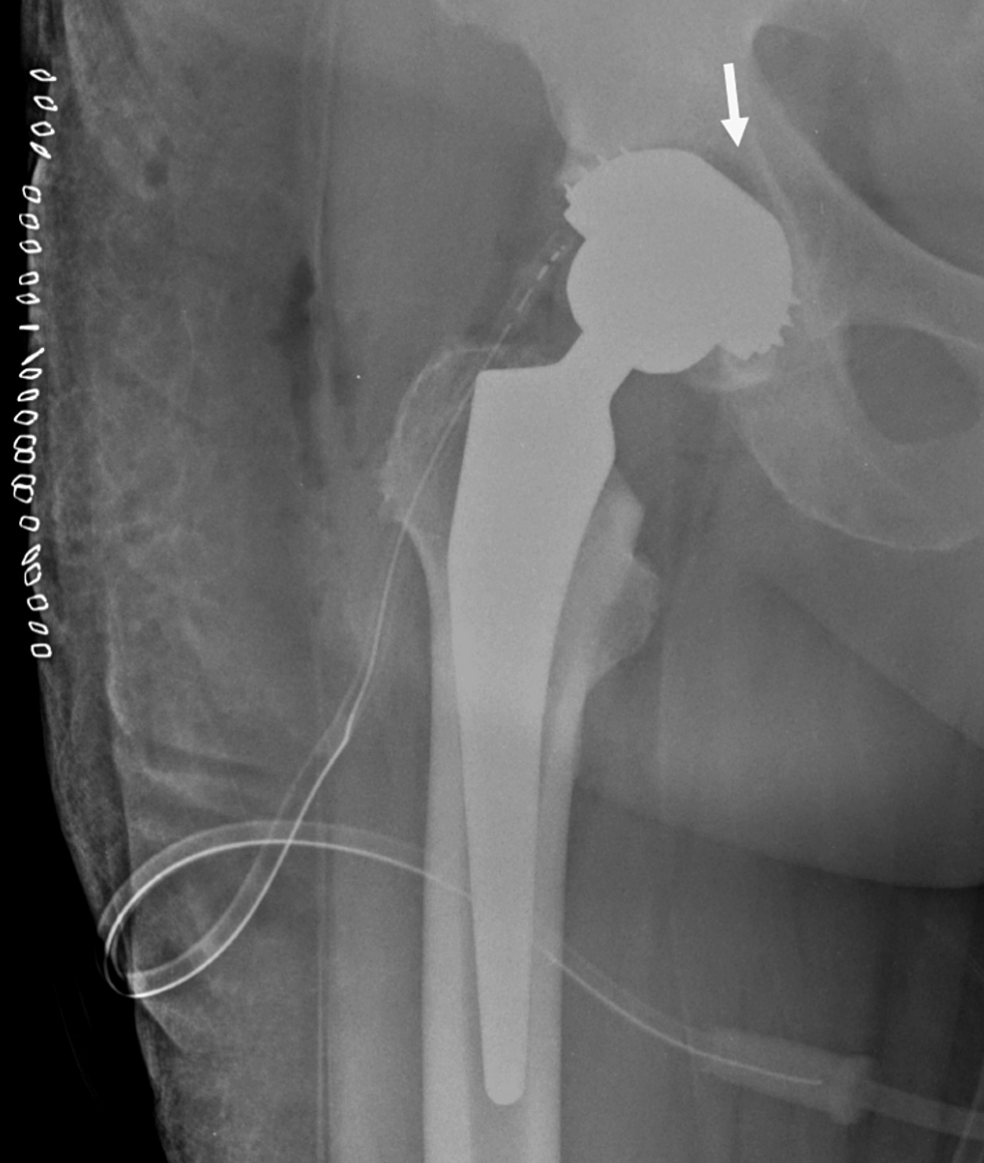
Anteroposterior postoperative X-ray view with the use of the EcoFit® SC threaded cup The white arrow shows the small distance between the acetabulum component and the bone bed
